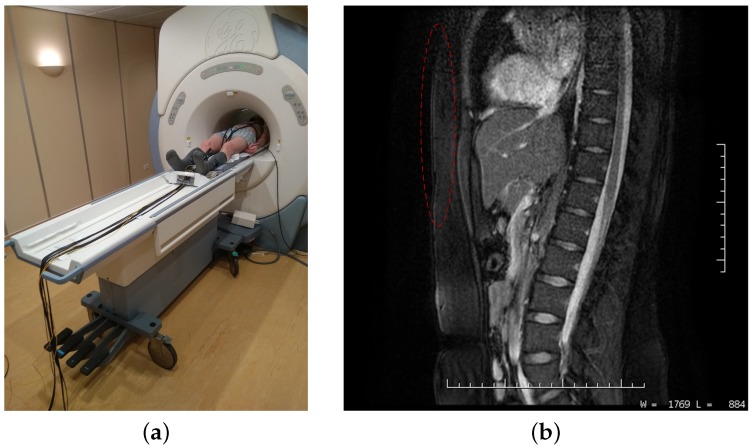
(a) Photography from the MRI examination; (b) MRI image acquired from the sensor location: sagittal view—test subject M2 (512 × 512 resolution).
